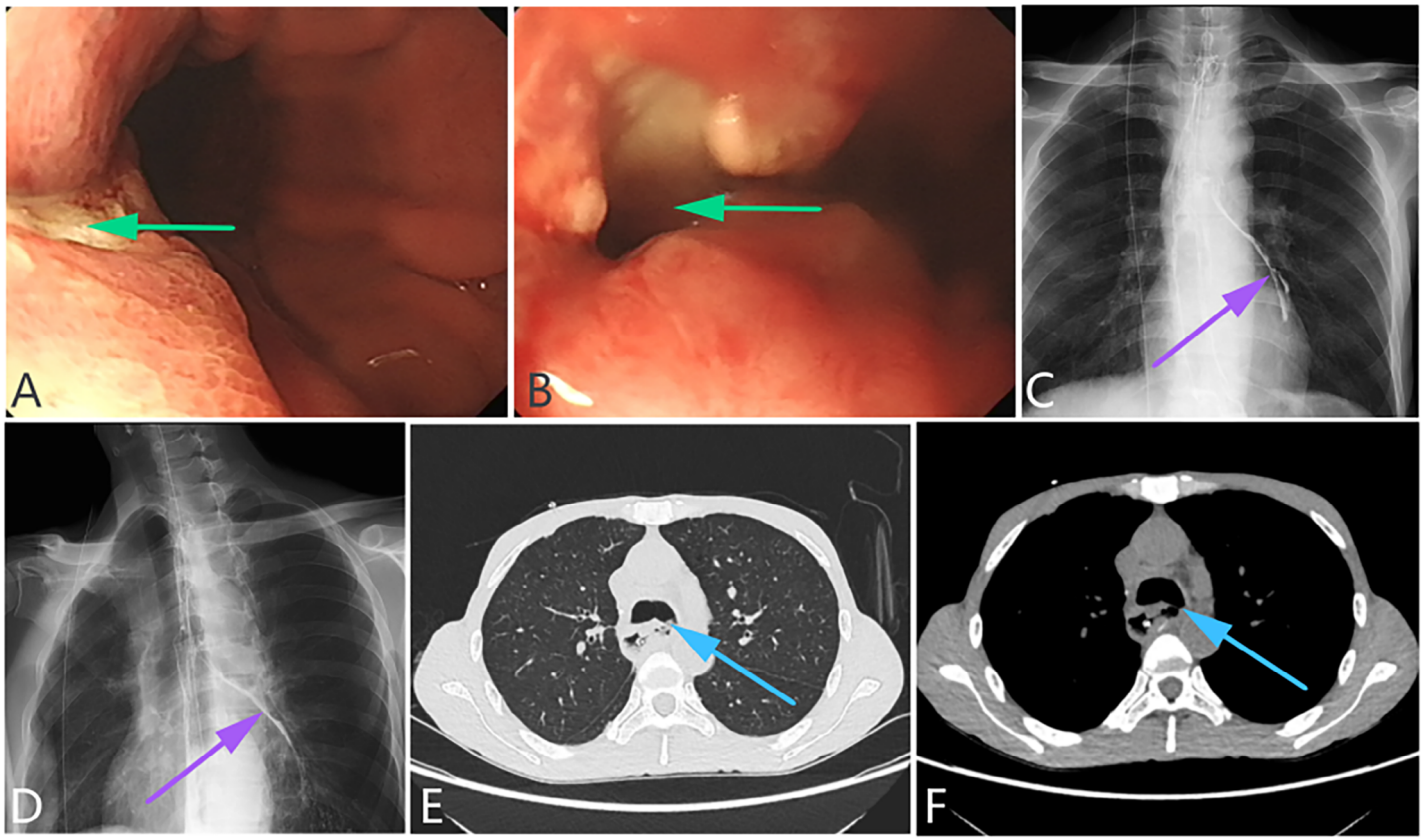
Postoperative anastomotic recurrence of esophageal malignant tumors leads to fistula formation. A, B) After surgery for malignant esophageal tumors, the anastomotic site recurred, and a fistula formed locally. C, D) Upper gastrointestinal image showing a fistula and left bronchial image. E, F) CT scan showing a pathway between the anastomotic site and the left main bronchus. (Original work created by the authors).
